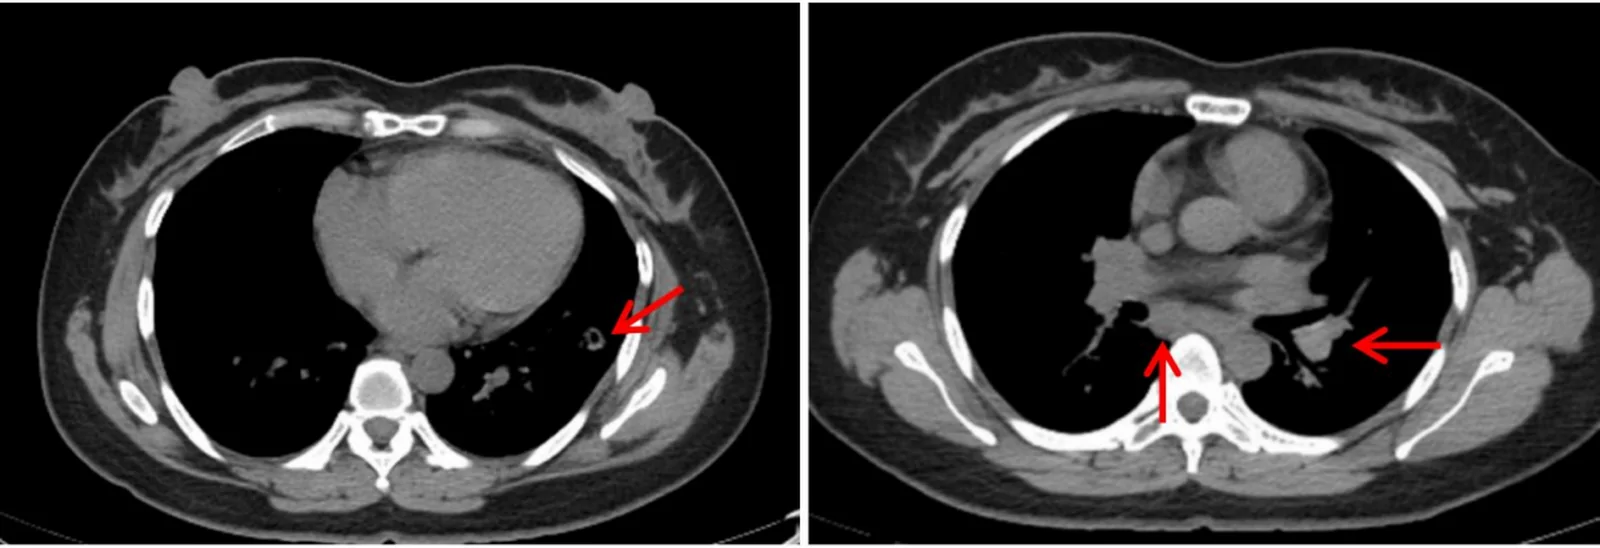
Baseline CT before the inititation of neoadjuvant lorlatinib. Chest CT revealed a nodule in the left lower lobe with hilar and mediastinal lymphadenopathy. CT, computed tomography
